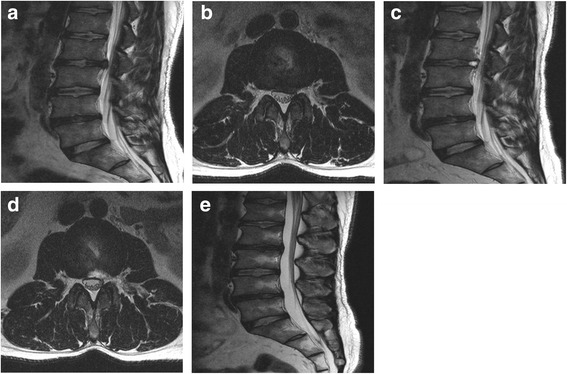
One year later, MR images showing left paramedian disc herniation at the L2–3 (a and b). After the PELD, MR images showing complete removal of the disc (c and d). Eighteen months later after second PELD, MR image (e) showing no significant changes of the disc height or any disc degeneration aggravation in comparison with initial MR images
